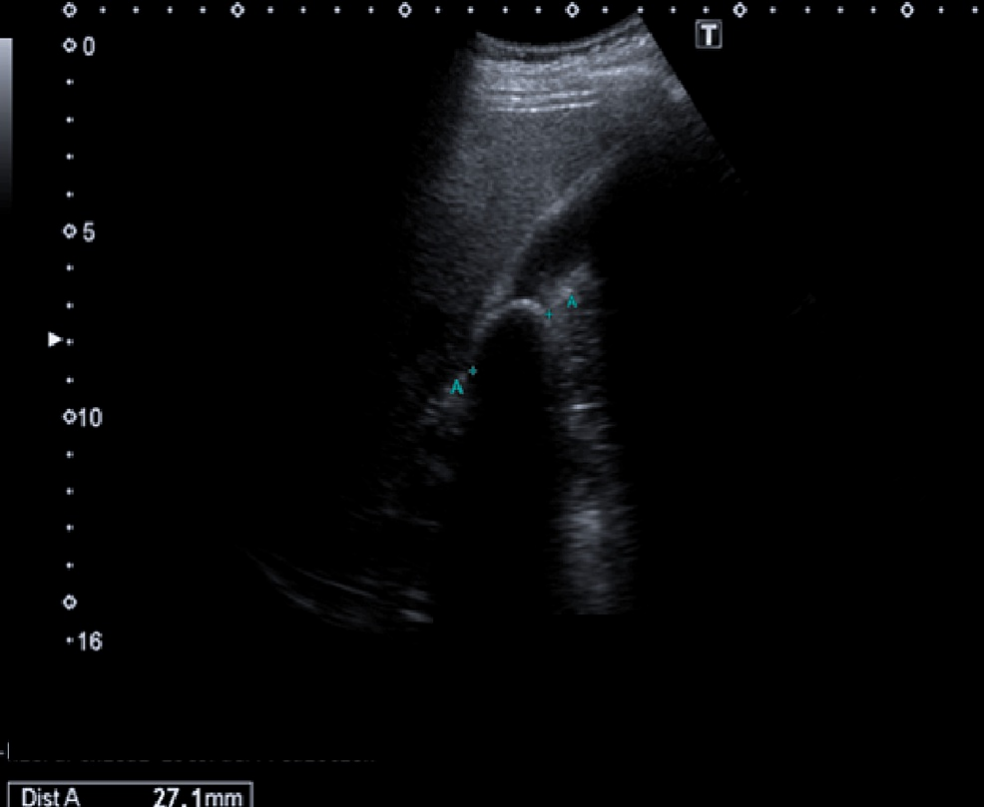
Abdominal ultrasound that confirms the diagnosis of cholelithiasis, revealing a 27-mm gallstone within the gallbladder. No radiological signs of acute cholecystitis were evident during the study. The condition of situs inversus was reaffirmed by the radiologist as an added comment on his ultrasonographic report, as he added the following note: "Patient with a history of SIT, so the liver and gallbladder are visualized in the upper left hemiabdomen, and the spleen in the upper right hemiabdomen."
